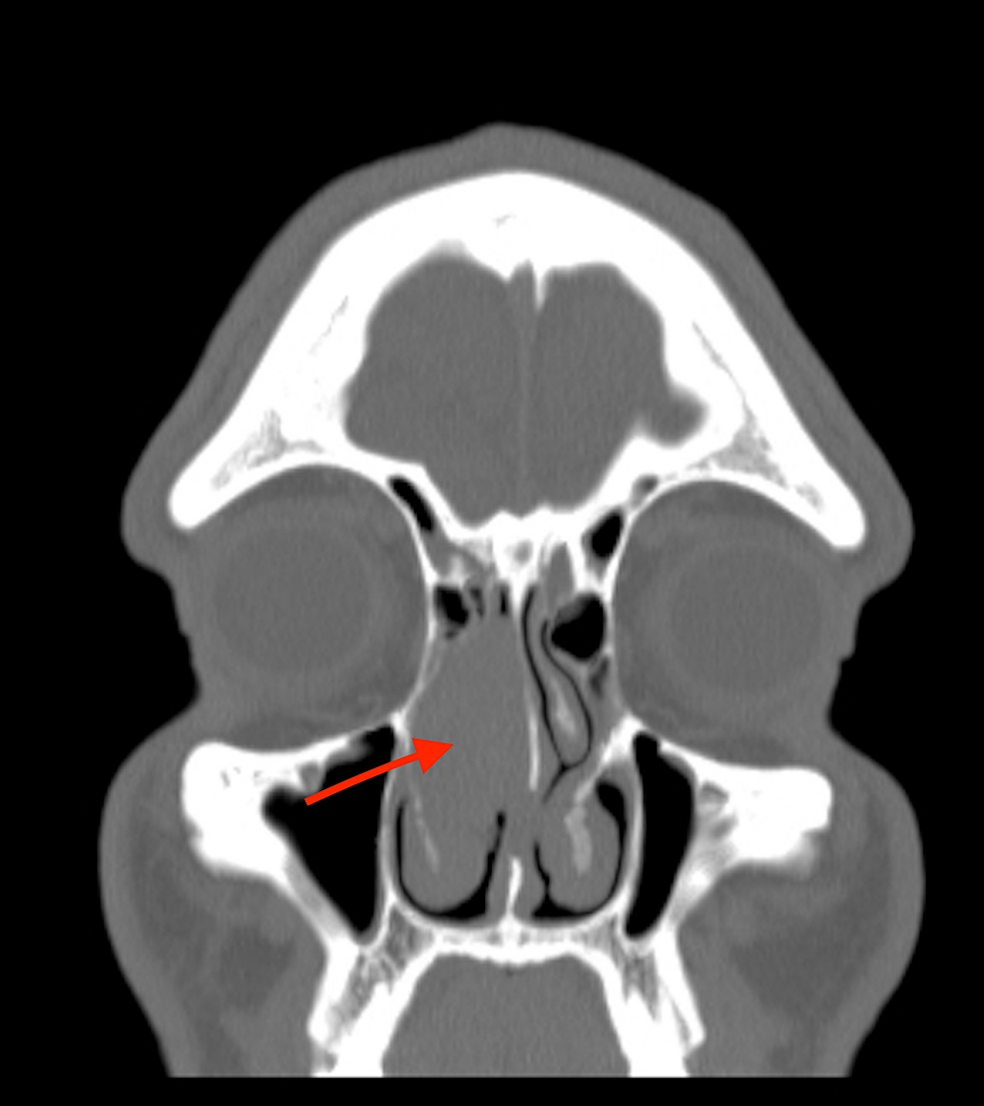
Coronal soft tissue contrast-enhanced computed tomography scan of the paranasal sinuses and orbit
